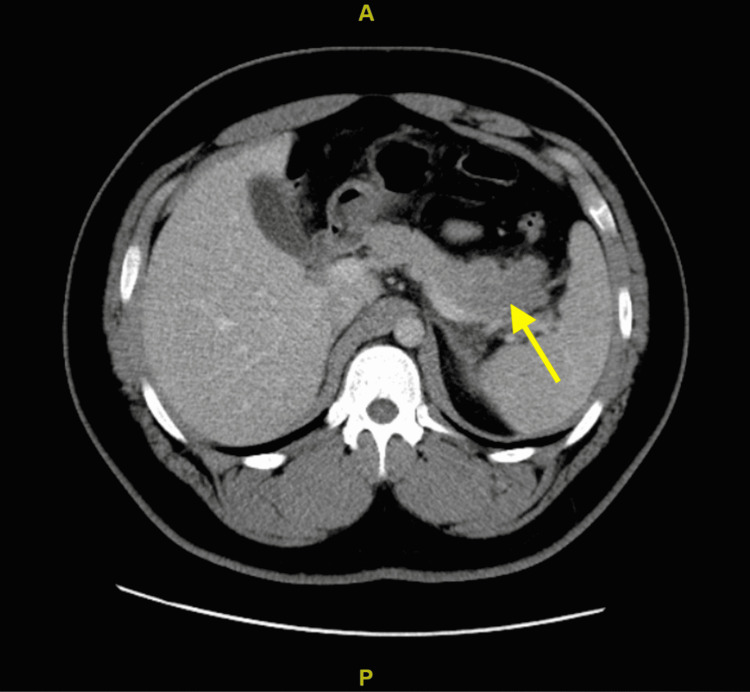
Pancreatic Tail Injury on CT Imaging
